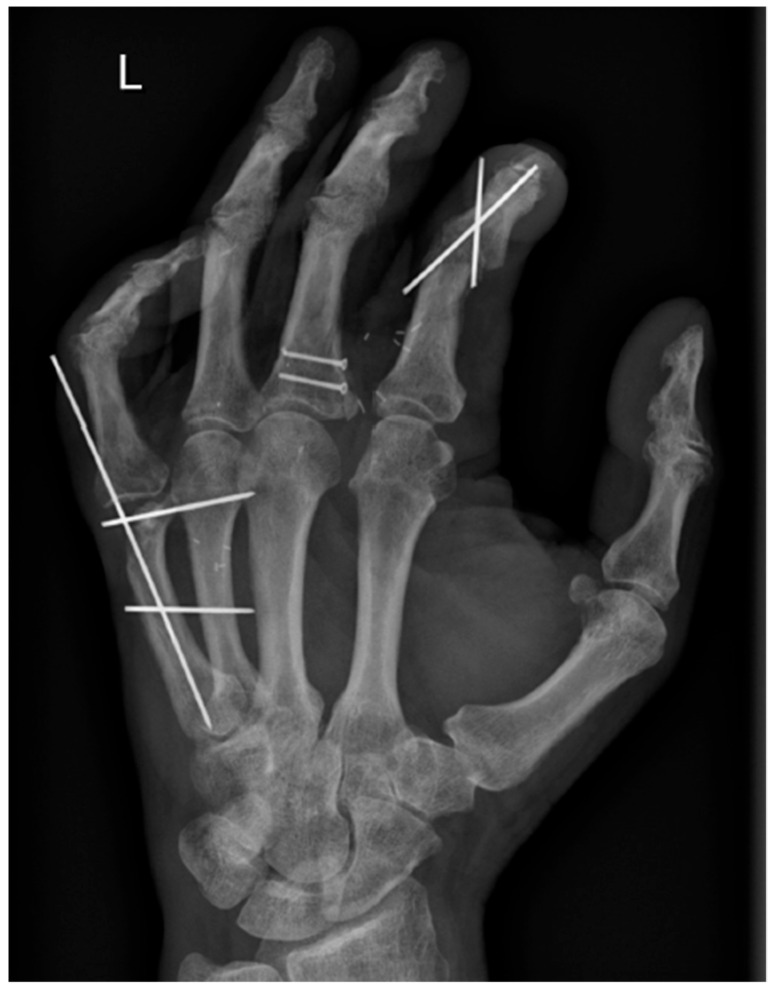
Postoperative X-ray image of the injured hand.
